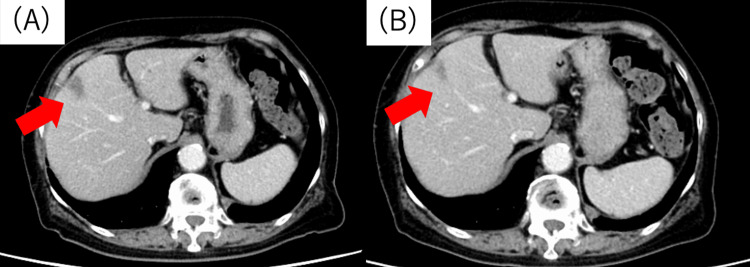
Findings of follow-up CT during hospitalization at our hospital. Contrast-enhanced CT showed that the abscess in S4 gradually shrank (arrow) (A) on day 1 (19 mm) and (B) on day 28 (13 mm) CT: computed tomography
